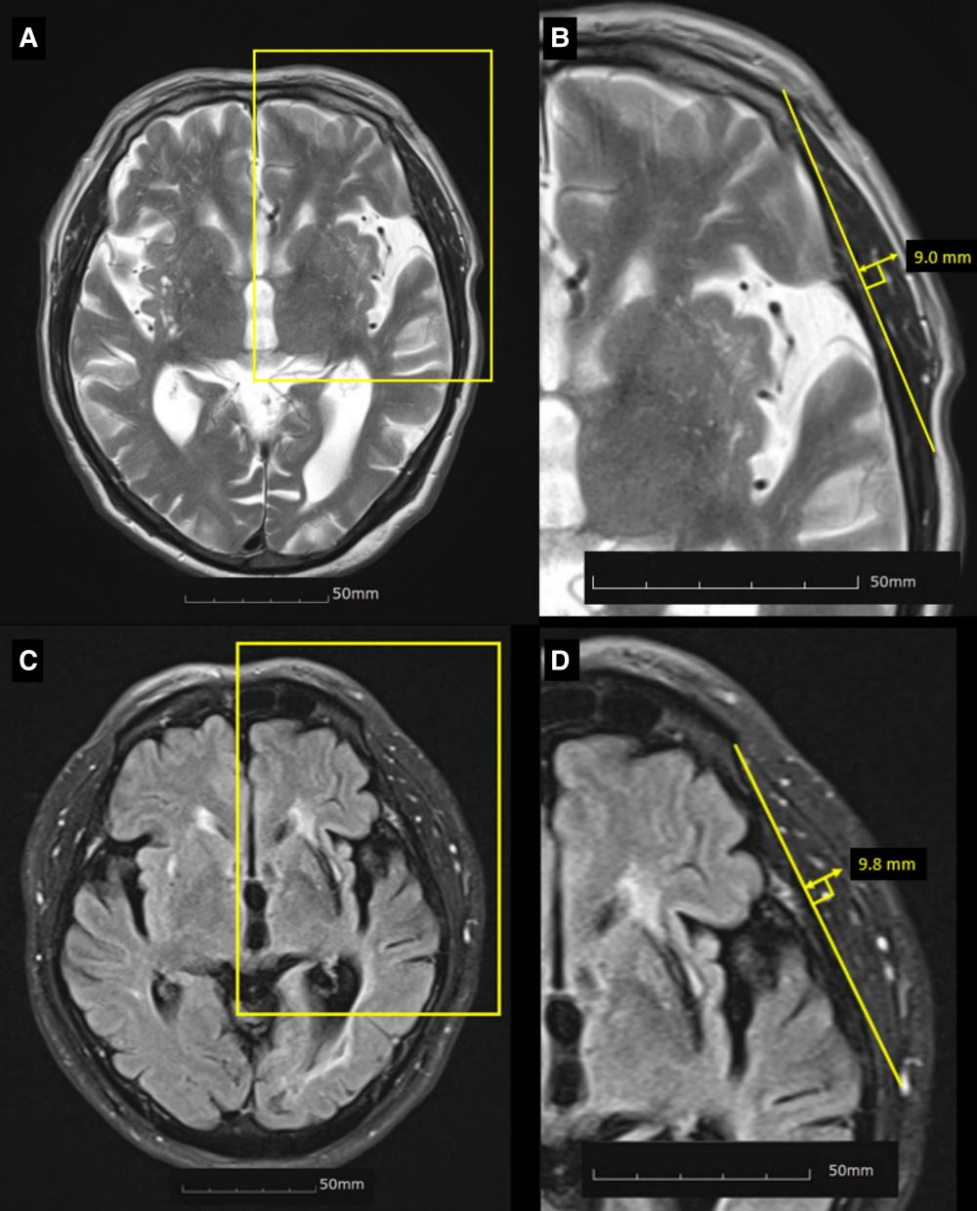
Measuring TMT. TMT was measured on the axial plane of either (A and B) T2-weighted or (C and D) FLAIR images, which were oriented parallel to the anterior commissure–posterior commissure line. (A, C) TMT was assessed at the orbital roof level. Enlarged views of the yellow square sections in (A) and (C) are shown in (B) and (D), respectively. (B, D) TMT was defined as the length perpendicular to the longitudinal axis of the temporal muscle. The Sylvian fissure was used as a reference point in the anterior–posterior orientation. TMT, temporal muscle thickness.
